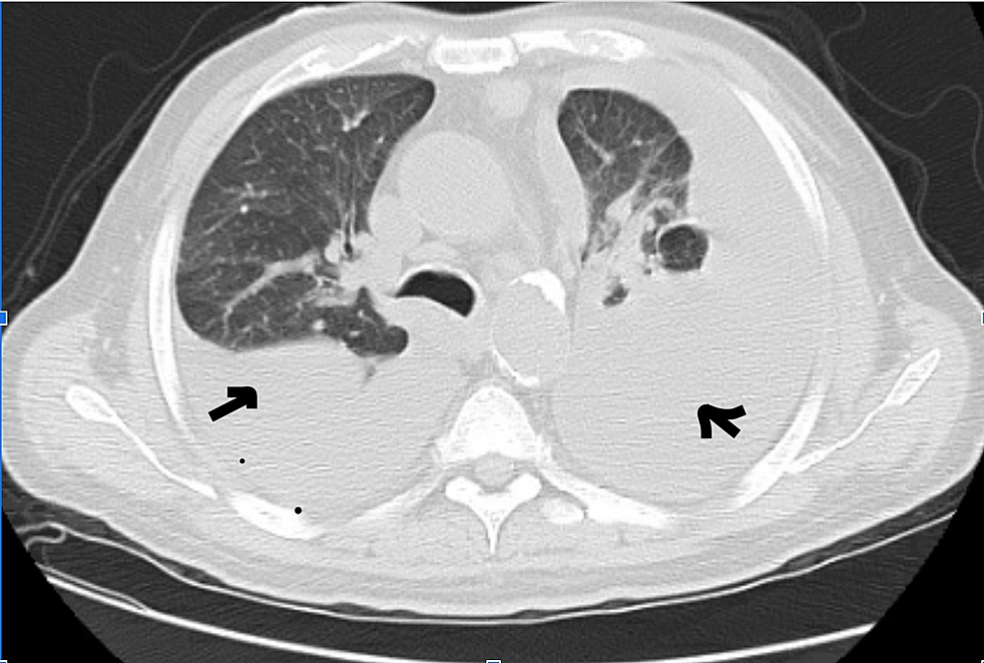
CT of the chest without contrast on admission showing bilateral pleural effusion (arrows) CT: computed tomography
